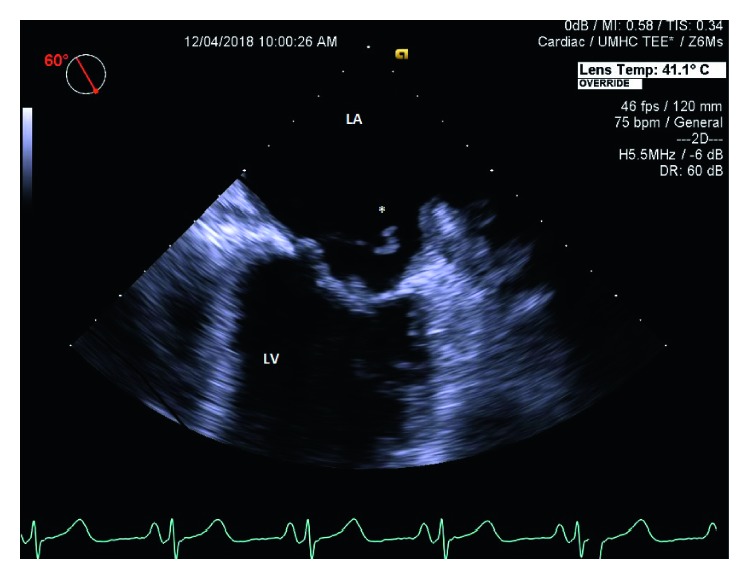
Transesophageal echocardiogram at the mid-esophageal level with 60 degree rotation. Ruptured chordae tendineae from the posterior mitral valve leaflet (marked with an asterisk).
